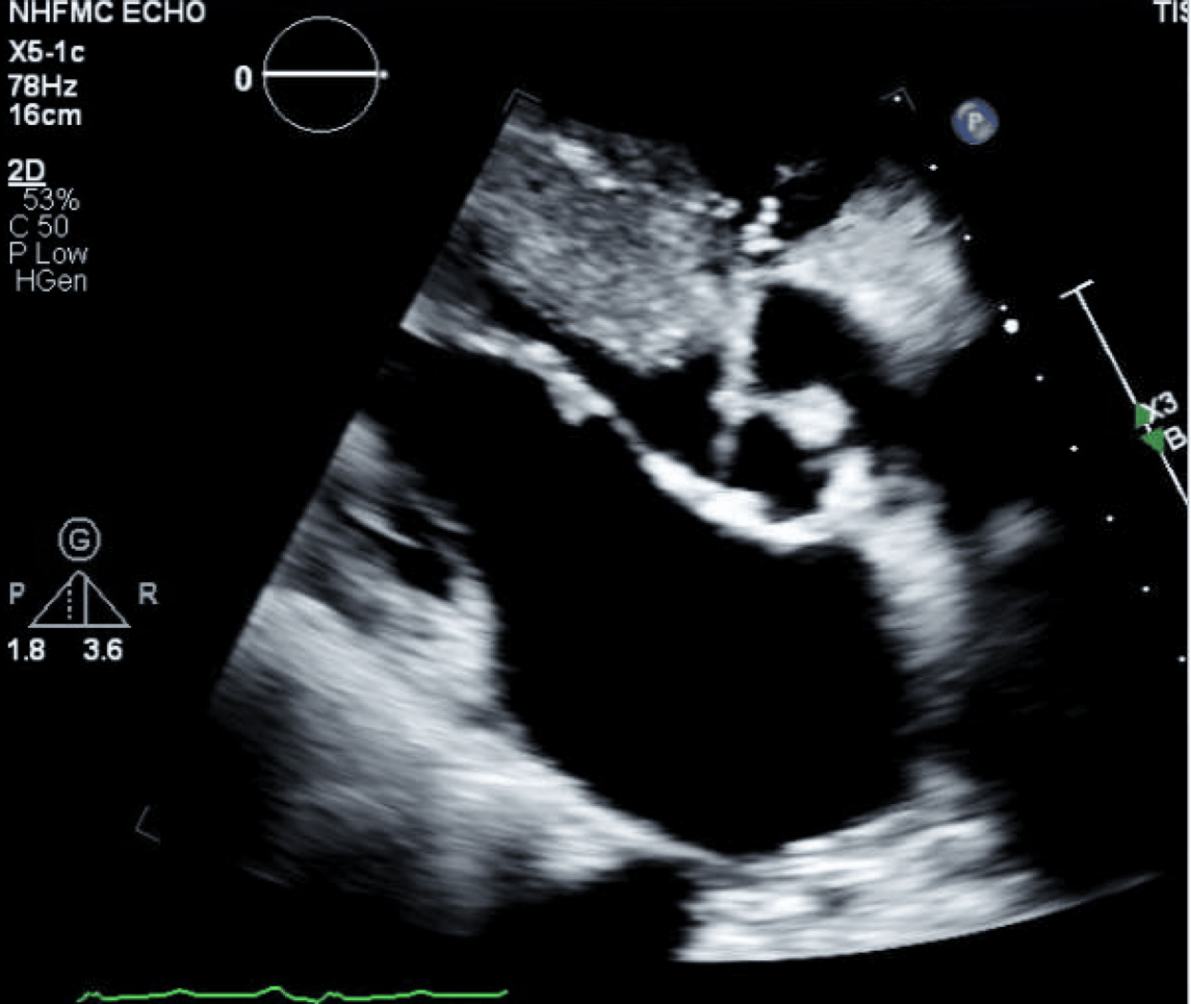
Transthoracic echocardiogram revealing a highly mobile, pedunculated mass attached to the aortic valve, consistent with a fibroelastoma. The lesion is obstructing the left ventricular outflow tract and displays a frond-like configuration.
